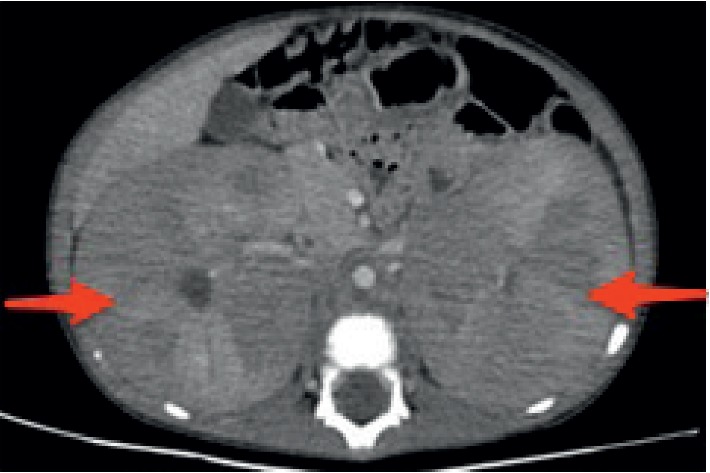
Axial CT image shows diffusely enlarged kidneys. Intestinal bowels and liver are compressed anteriorly.
